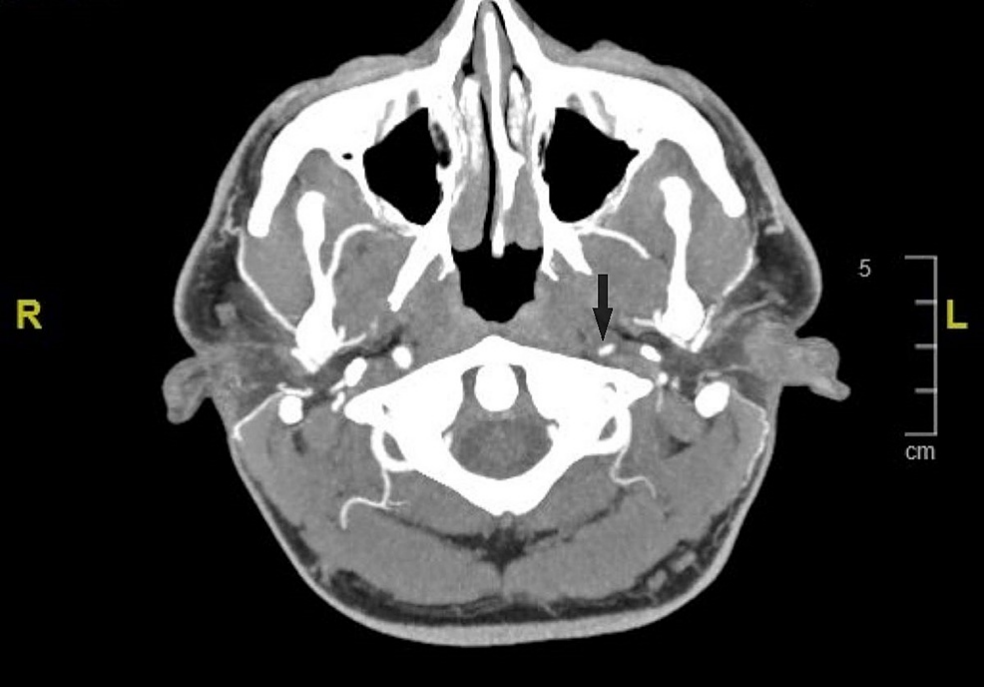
CT angiography of the head shows a high-grade luminal narrowing of mid and distal left internal carotid artery Arrow points to left internal carotid artery with 80% luminal narrowing.
